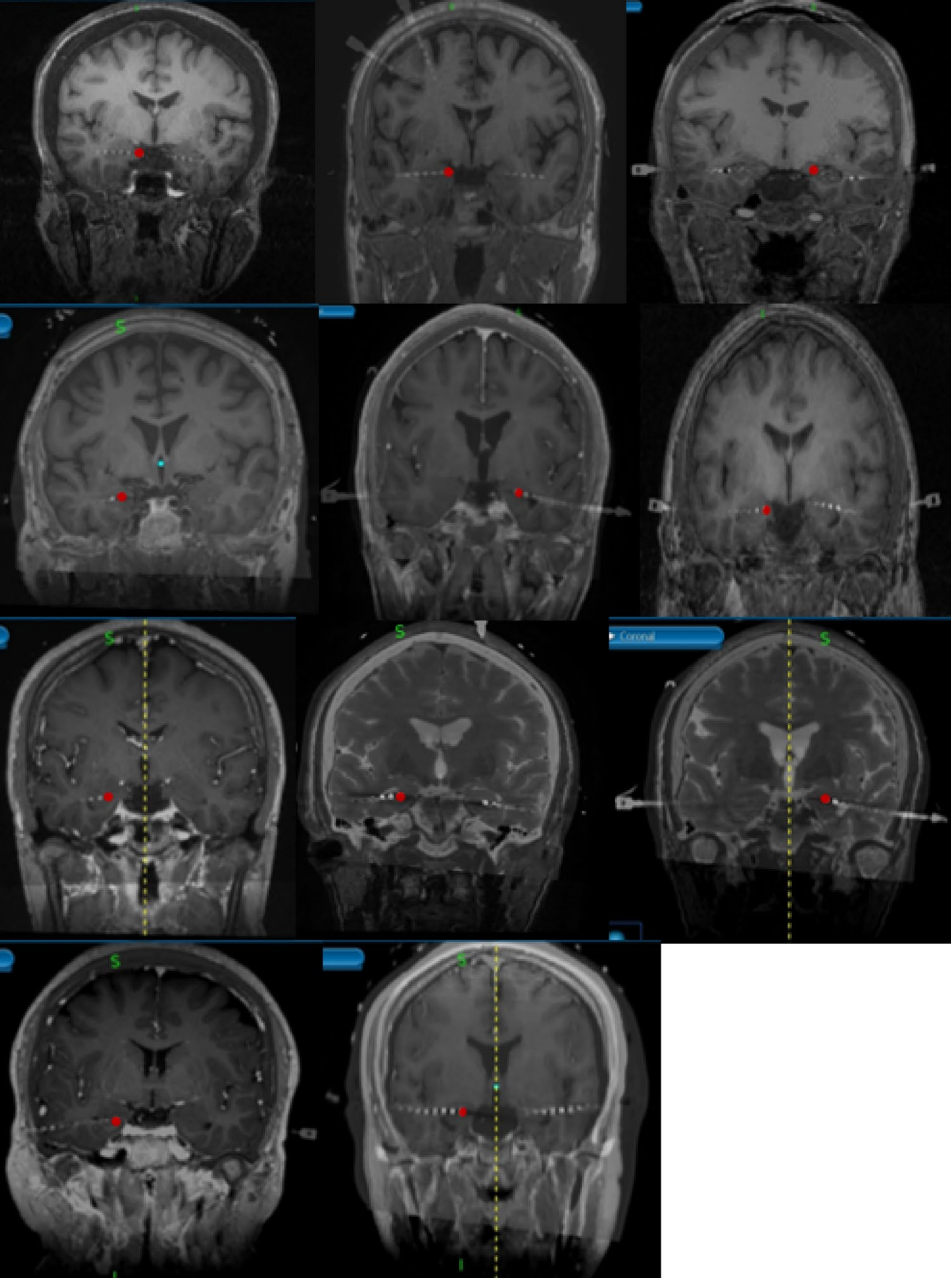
CT and MRI merged images using Medtronic StealthStation of 11 patients. The red dot in each figure represents the most distal hippocampus contact in the electrode
